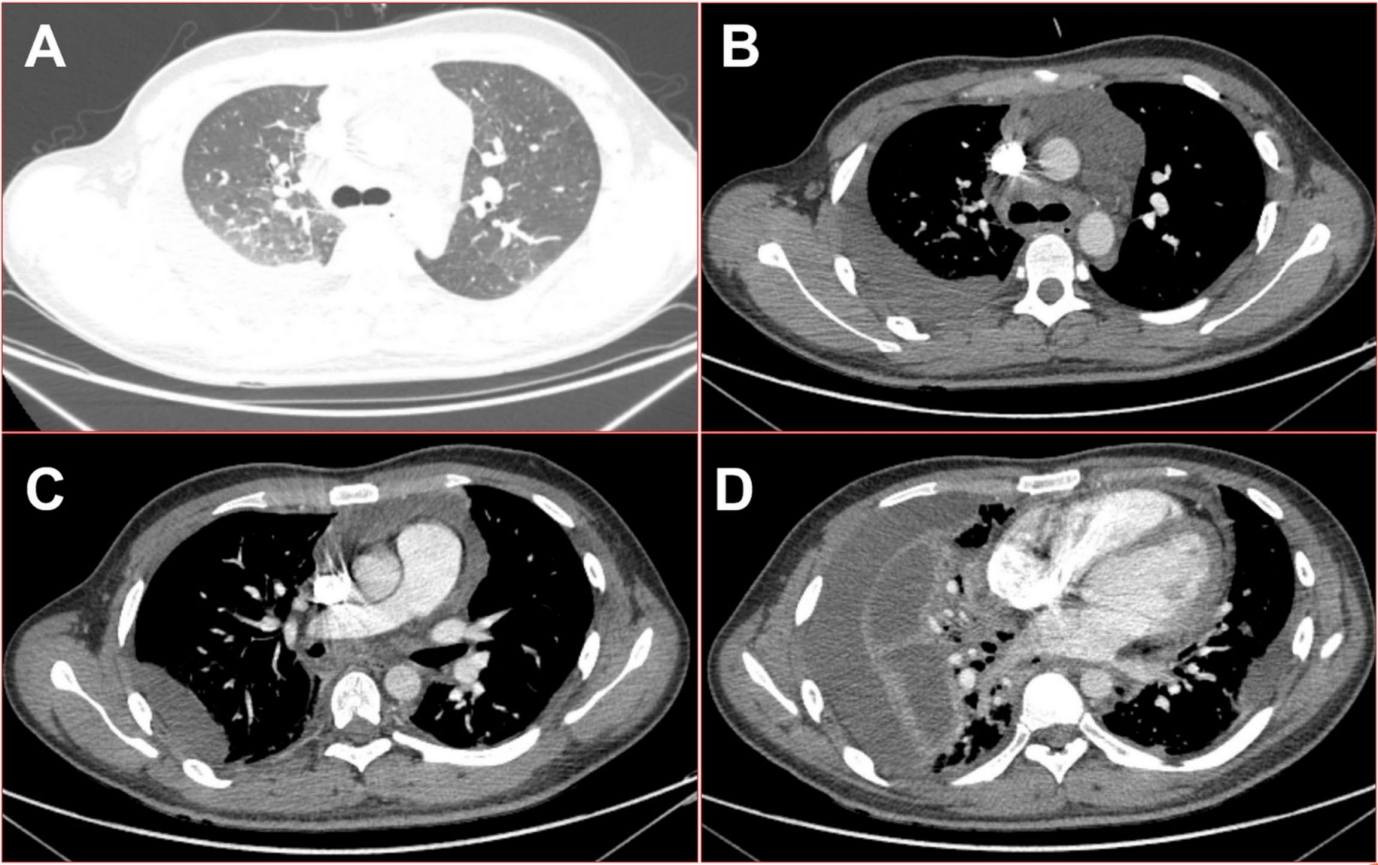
On the seventh day of the fever, 16-slice computed tomography images of the patient’s chest revealed multiple serous cavity effusions. (A) Lung window in the upper thoracic cavity; (B) mediation window in the upper thoracic cavity; (C) mediation window in the middle part of the thoracic cavity; (D) mediation window in the lower thoracic cavity.
